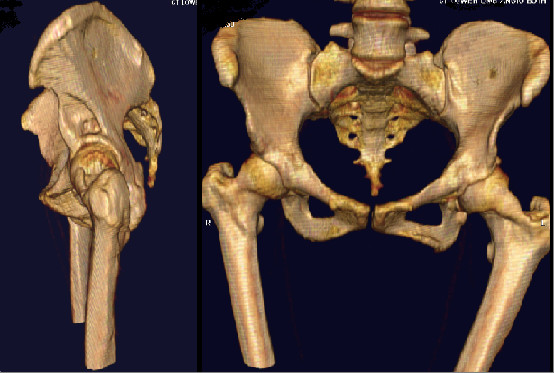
CT reconstructions demonstrating erosion of left femoral neck and pelvis.
